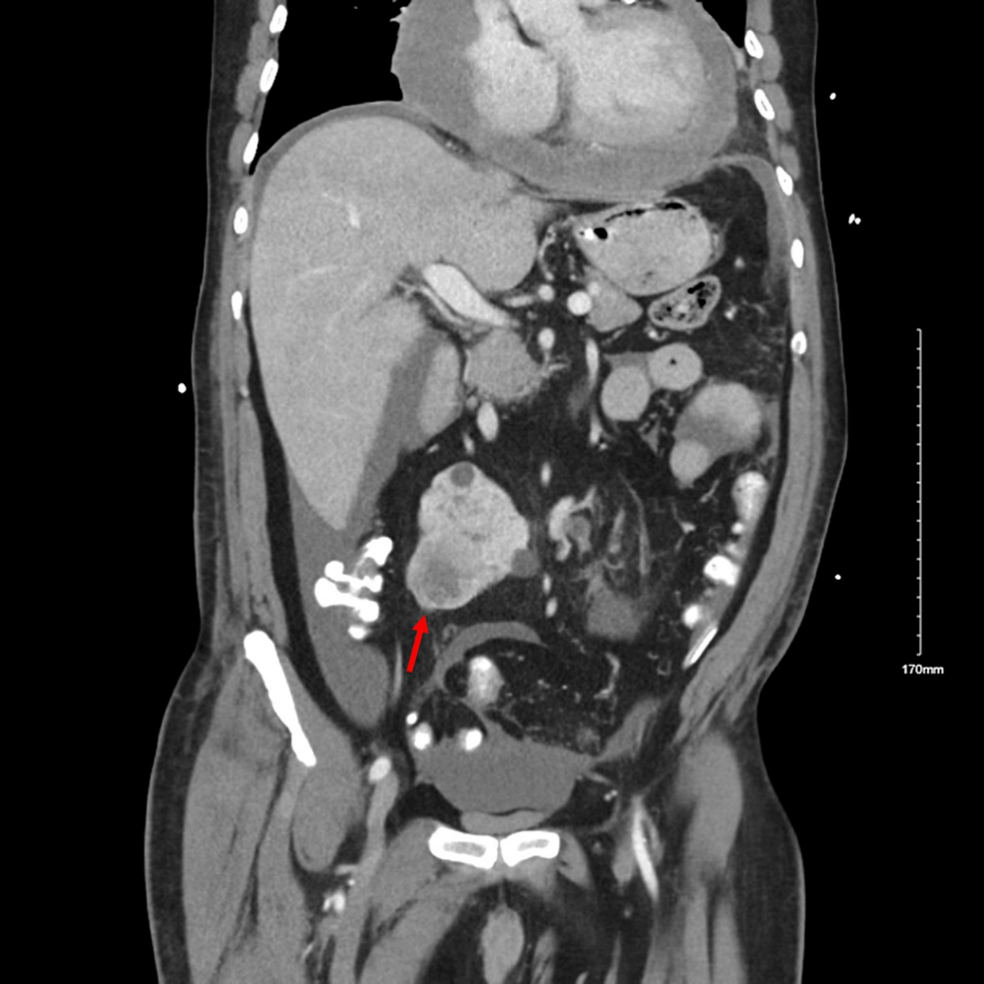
Sagittal CT abdomen/pelvis shows a 4 cm rounded heterogenous mass with some internal necrosis in the right kidney (red arrow). CT: computed tomography
